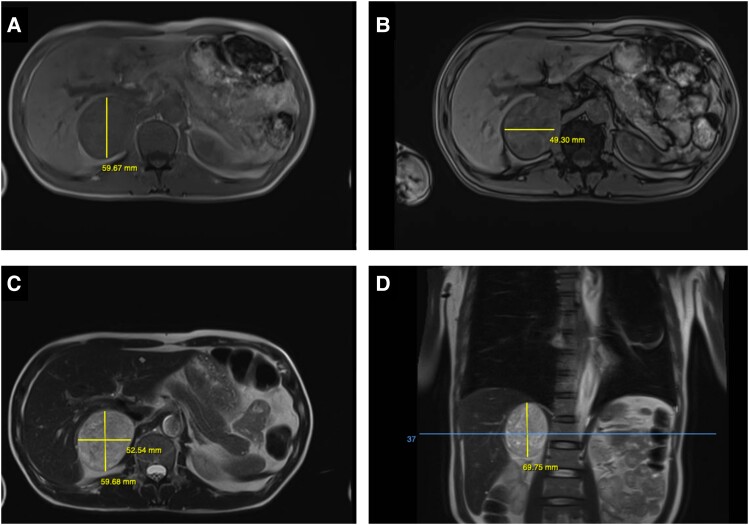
Abdominal MRI images demonstrating a well-defined right adrenal tumor with a heterogenous soft tissue density shadow. A and B, Axial T1-weighted images showed a mass measured 6 × 5 cm with low signal intensity. C, Axial T2-weighted image and D, coronal T2-weighted image showed increased signal intensity.
